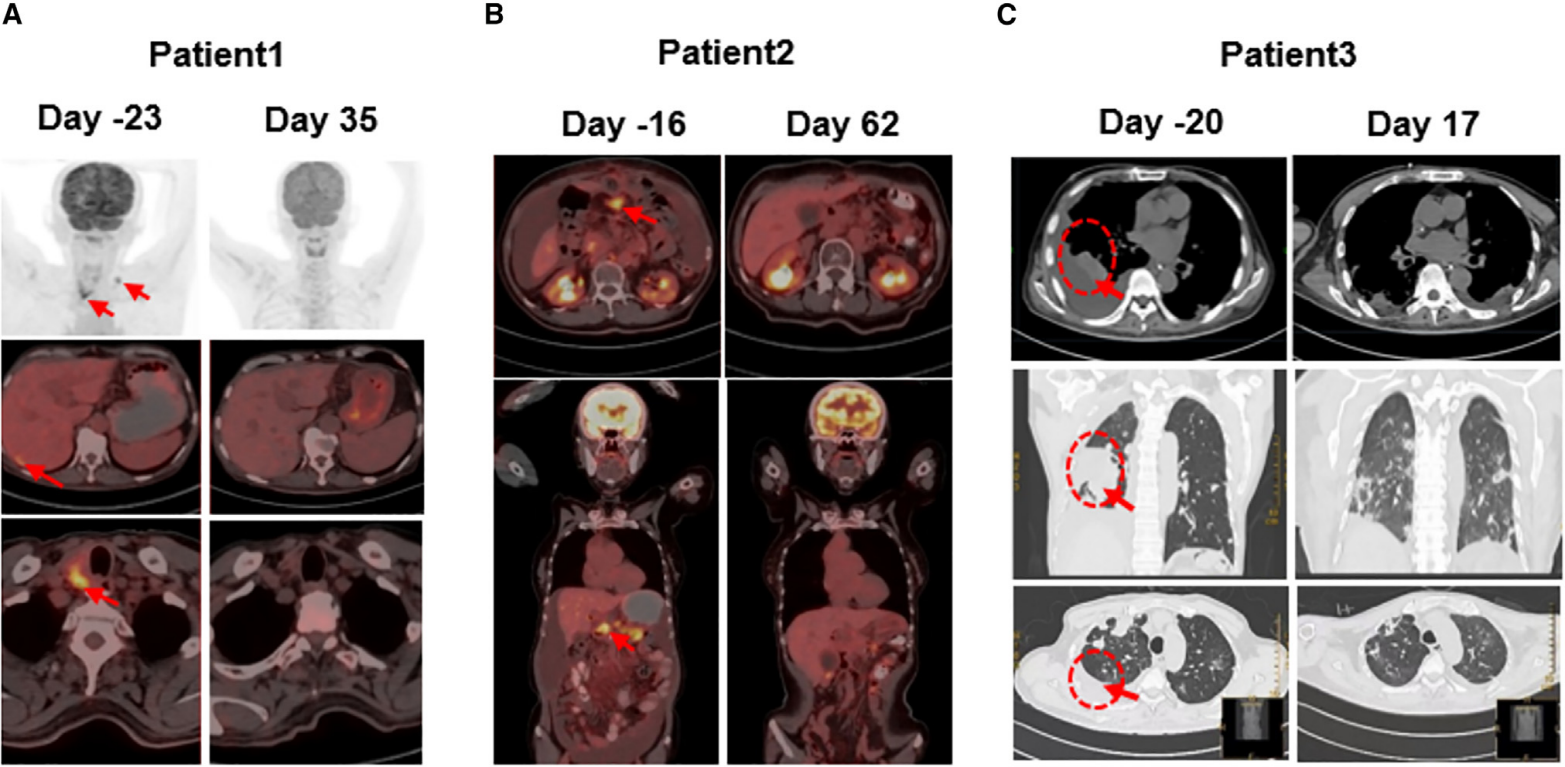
Evaluation of treatment efficacy (A) PET-CT shows that the lymph node metastases in zones III and V of the neck disappeared 35 days after CAR-T cell infusion in patient 1, as well as the right posterior lobe of the liver or intrahepatic metastatic lesions. (B) PET-CT shows that the recurrent lesion at the surgical resection site was eliminated 62 days after CAR-T cell infusion in patient 2. (C) CT scan shows the disappearance of pulmonary metastases and pleural effusion 17 days after CAR-T cell infusion in patient 3.
